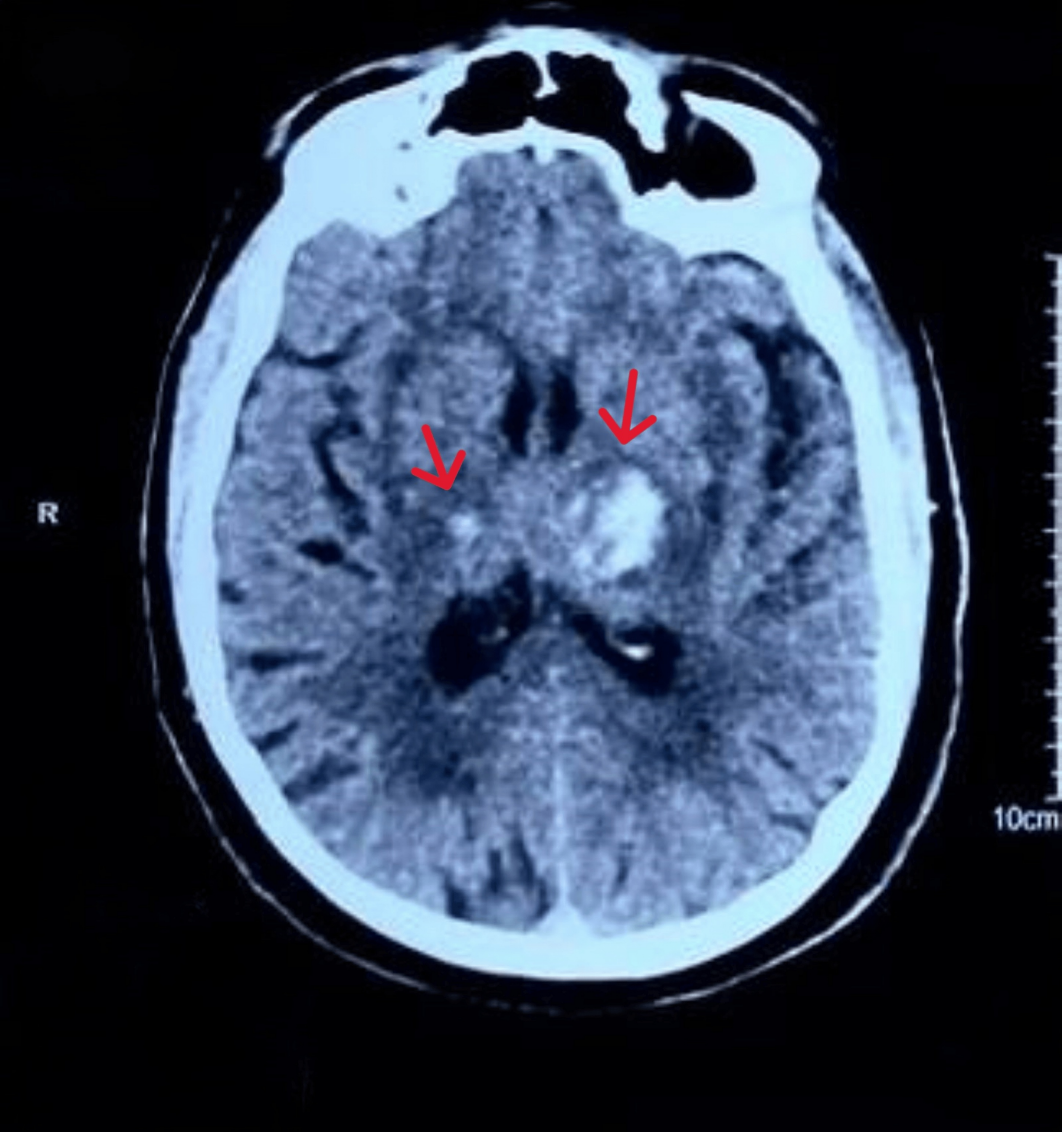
A CT scan of the brain (axial view) shows the presence of bilateral hyperdensities at the basal ganglia area (marked by red arrows).
